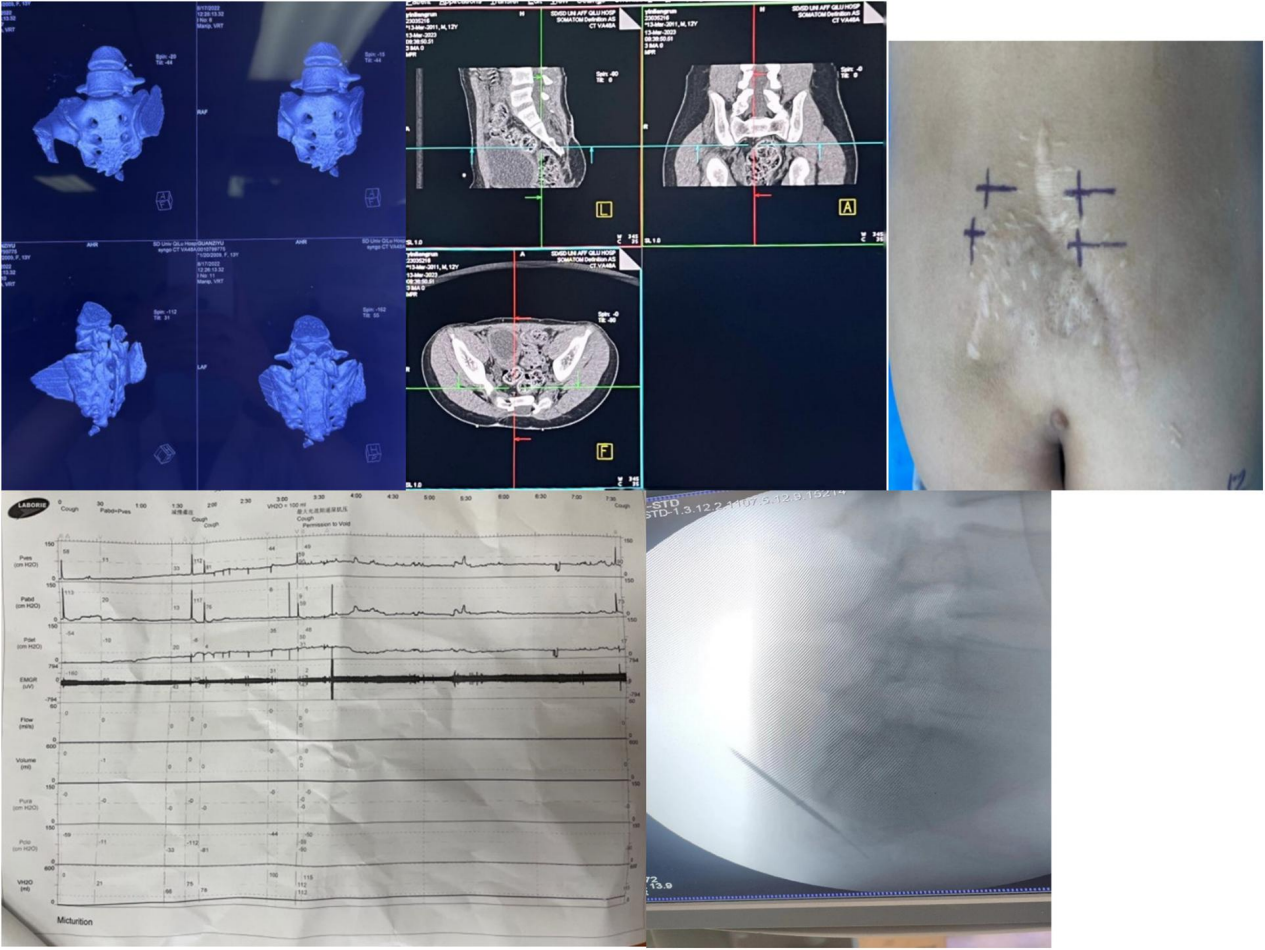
Preoperative localization, baseline urodynamic assessment, and intraoperative electrode placement for sacral neuromodulation (SNM). (a) Preoperative sacrococcygeal three-dimensional reconstruction is performed to identify bony deformities and exclude surgical contraindications. (b) Computed tomography (CT)–based localization of the S3 foramen facilitates accurate puncture and reduces operative time. (c) The body surface puncture site is marked according to CT landmarks, and alternative puncture sites (e.g., contralateral S3, S4, or S2) are prepared in case of inadequate response at S3. (d) Baseline urodynamic testing is conducted to evaluate bladder function prior to implantation. (e) Intraoperative C-arm fluoroscopy confirms needle entry into the S3 foramen, guides puncture depth, and assists in optimal electrode positioning while ensuring procedural safety.
